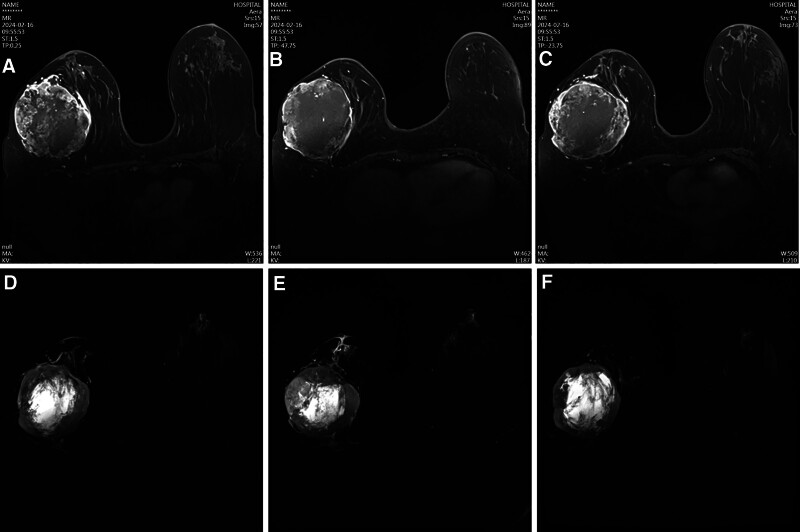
(A–F) MRI of the right breast tumor when the patient’s diagnosis. MRI = magnetic resonance imaging.
